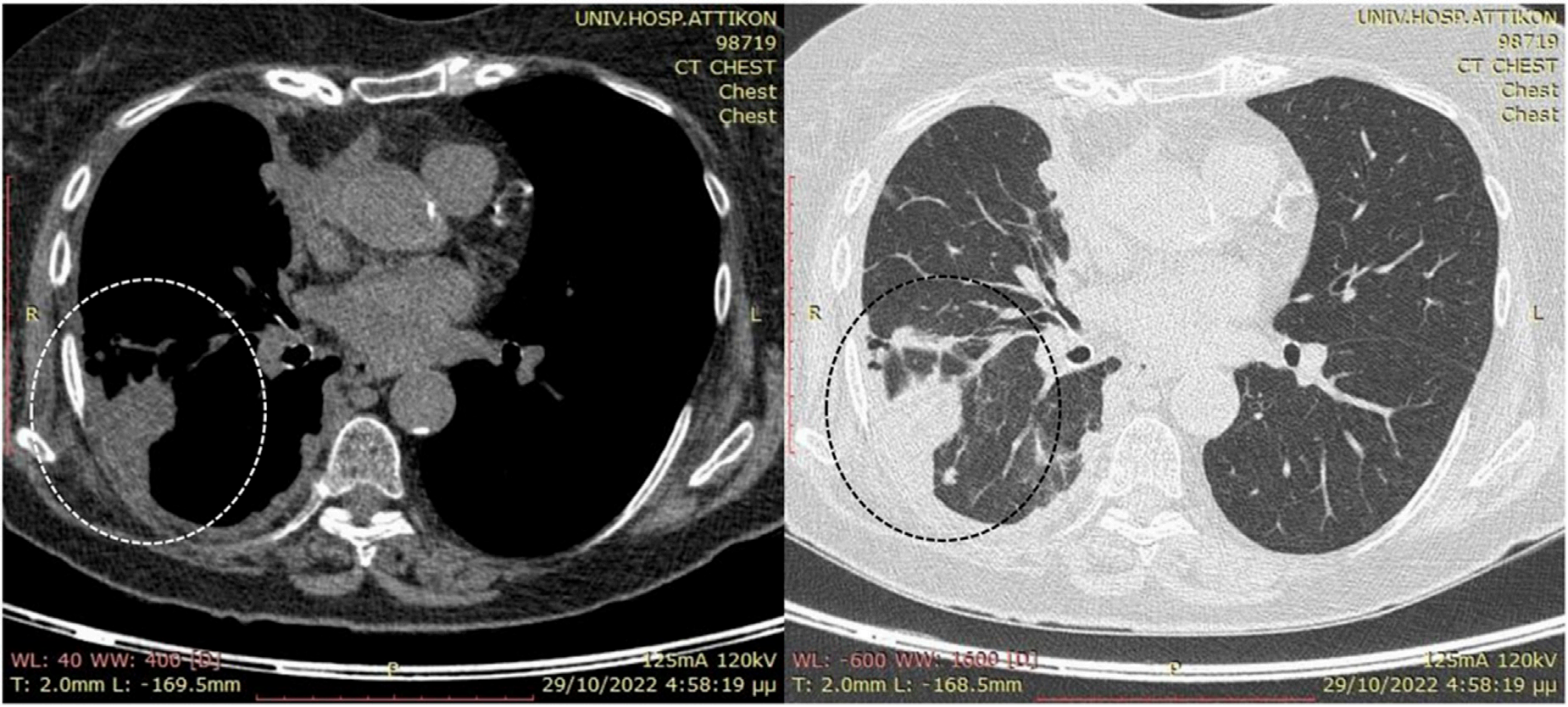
Preoperative computed tomography (CT) scans without intravenous contrast due to suspicion of renal disease. Note the presence of the large right-side pleural effusion (enclosed by the dotted circle) along with localized nodularity at the posterior costal arches and limited pleural fluid in the interlobar fissure after percutaneous drainage.
